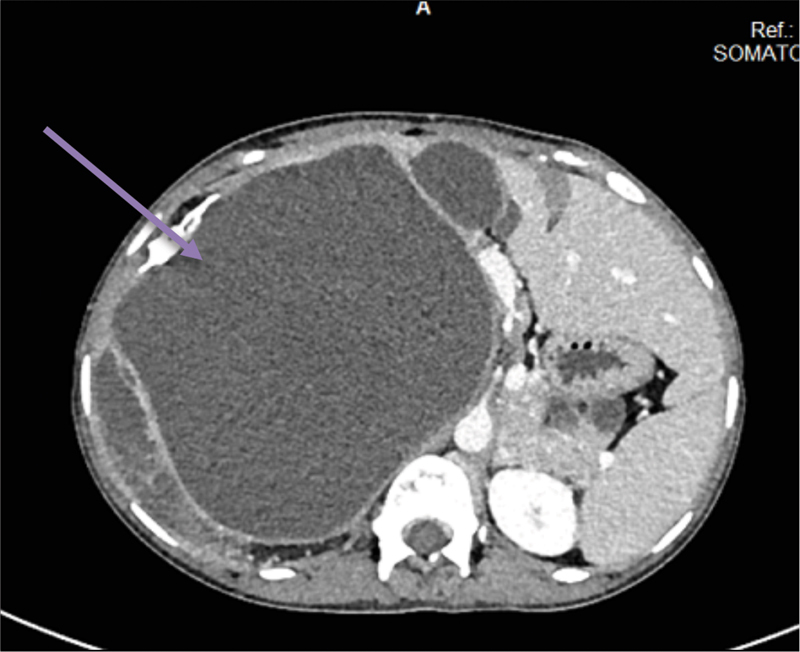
Contrast-enhanced computed tomography abdomen showing a large right-sided retroperitoneal mass compressing the right kidney and liver.
